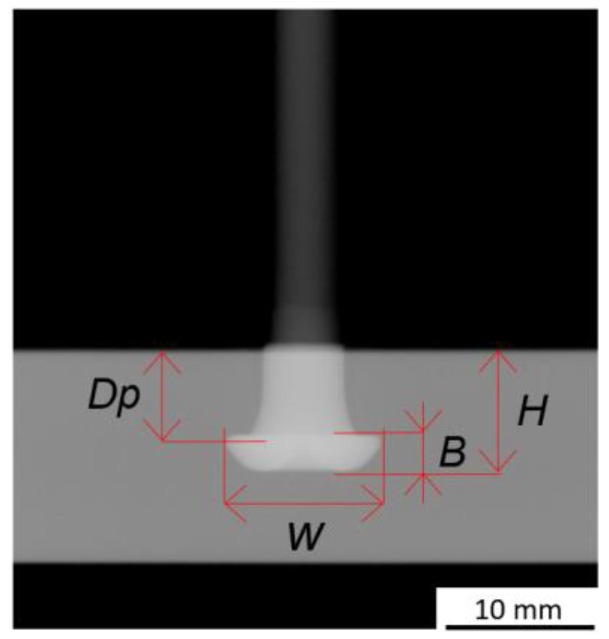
Example of X-ray tomography measurements for the joint formation analysis.
